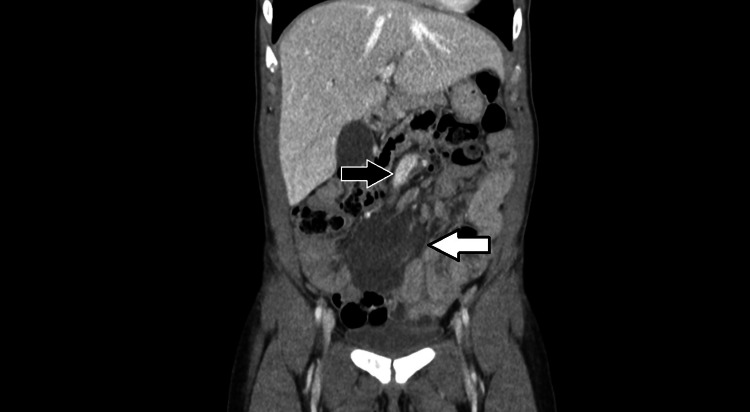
A coronal CT image showing a lobulated well-defined low-density mass with a number of prominent vessels that extend into the matrix of the lesion (white arrow). Congestion of the superior mesenteric vein (SMV) due to compression can also be observed (black arrow).
